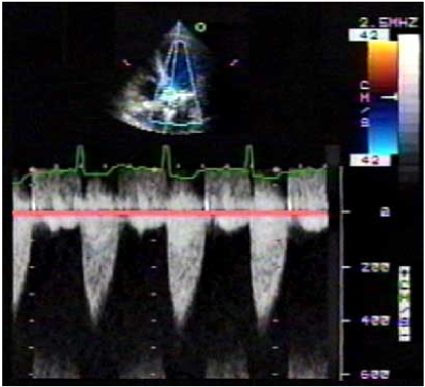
2-dimensional echocardiography reveals a calcified aortic valve with a max velocity of 4.4 m/sec, a mean velocity of 3.4 m/sec, a max gradient of 73 mm Hg with a mean gradient of 47 mm Hg, and an aortic valve area of 0.7 cm2.
